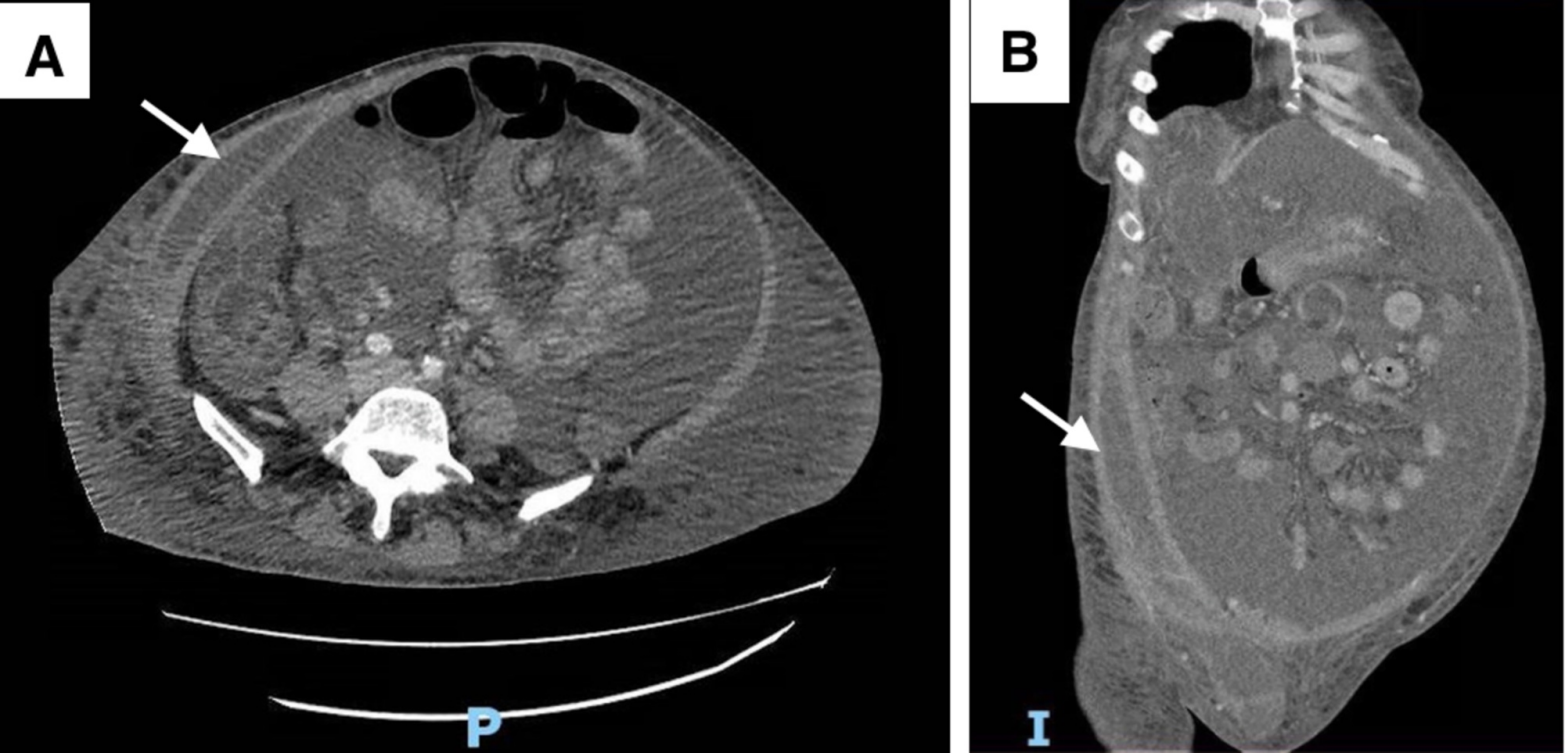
Axial (A) and Coronal view (B) of contrast-enhanced abdominal CT scan showing decreased size and continued evolution of right anterior abdominal wall hematoma status post coil embolization of the right inferior epigastric artery without any evidence of active bleeding.
